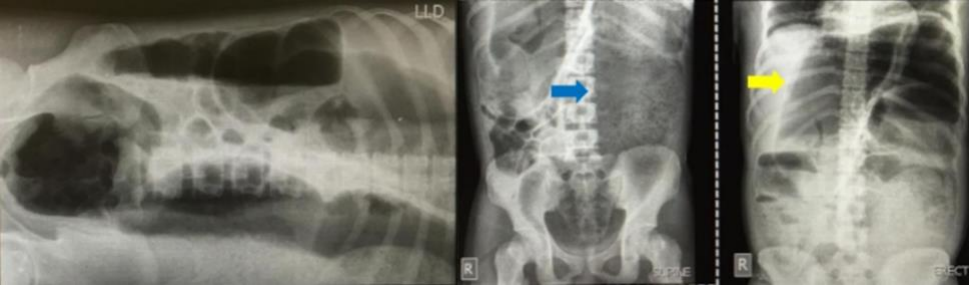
an X-ray of plain abdomen 3 views showed stool impaction (blue arrow) and dilated colon (yellow arrow); the colon diameter was more than 10 cm
